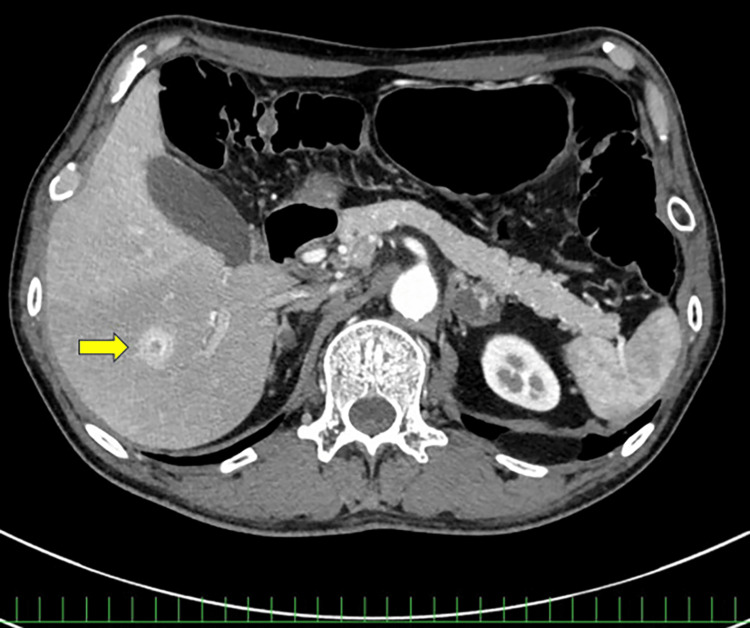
Baseline imaging of Case 2. Contrast-enhanced CT showing liver metastases (arrow).
